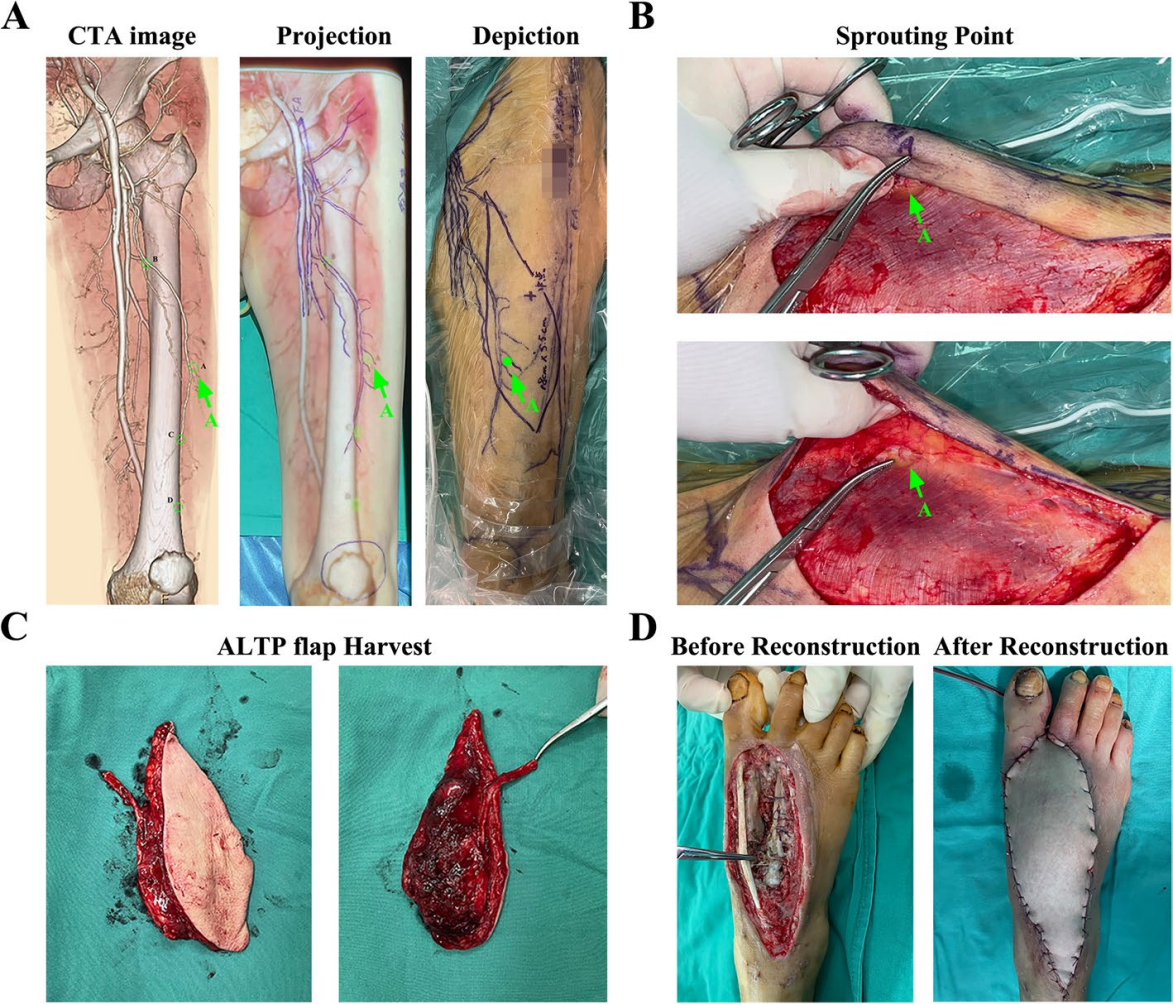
A typical case of a 56-year female. A Four marked emerging points A-D were showed in CTA images, but only one point was in the designed ALTP flap area. B During operation, the preoperatively marked point A was just above the emerging point of the perforator (green arrow). C The harvest ALTP flap and vascular pedicle. D After debridement, the damaged foot with 18.0 cm * 5.5 cm soft tissue defect was reconstructed by the ALTP flap
